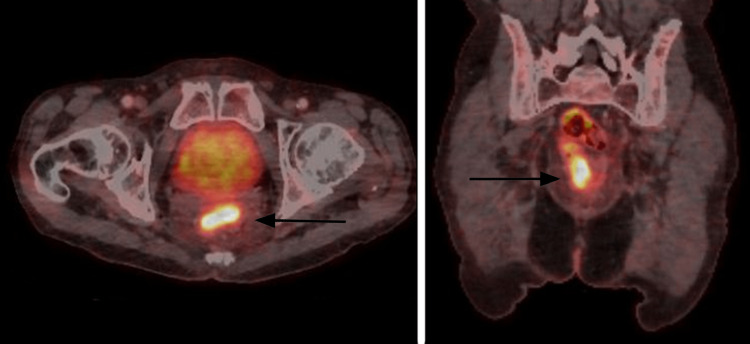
Axial and coronal reconstructions of a PET-CT exam centred on the pelvis showing high activity zones (black arrows) due to the inflammation caused by the caustic enema. PET-CT: positron emission tomography-computed tomography
